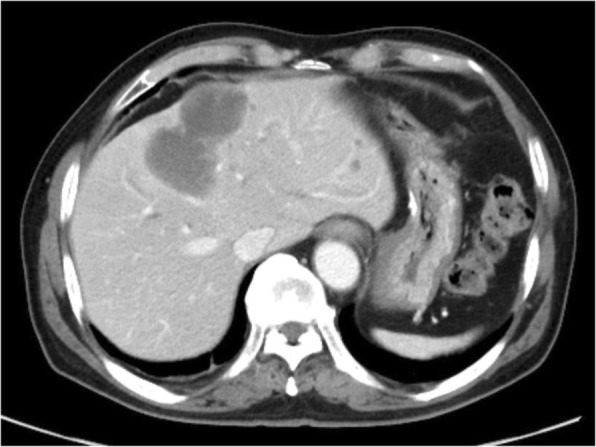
Enhanced computed tomography findings. A 40-mm-diameter tumor in hepatic segments 3 and 4 (S3+4) with enhanced solid nodule along the wall was detected. On the right side of the tumor, there was a 50-mm tumor, which suggested hemorrhagic cyst
